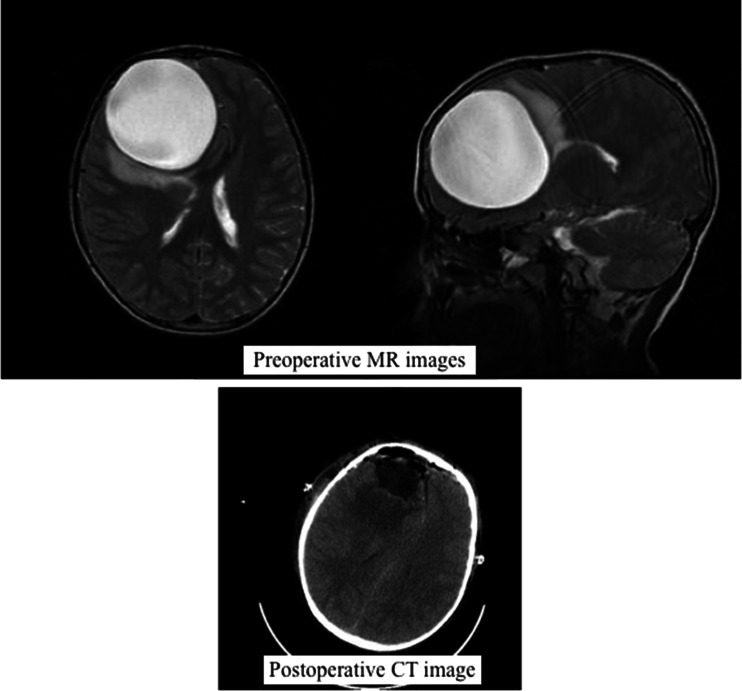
T2-weighted magnetic resonance (MR) image of a cystic lesion in accordance with a hydatid cyst, 7 cm in diameter, located in the right frontal lobe, exceeding the frontal cortex, with smooth edges, filled with homogeneous fluid and with shift and compression effects. Cranial CT check after removal of frontal hydatid cyst, showing reduction in cyst location and in compression effects of the cyst
